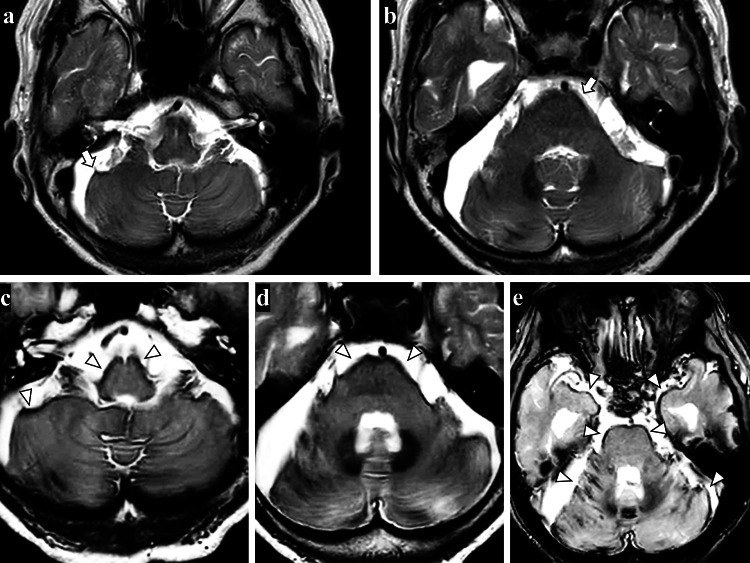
Pre- and postoperative brain MRI of the lumbar subarachnoid-peritoneal (L-P) shunt at the age of 78 years (a, b) and 80 years (c, d). (a, b) Preoperative axial T2-weighted MRI showing hemosiderin deposits on the surface of the brainstem and cerebellum (arrows); (c, d) At two years after L-P shunt surgery, T2-weighted MRI showing the increased deposition of hemosiderin (arrowhead); (e) T2*-weighted MRI showing numerous hemosiderin deposition (arrowheads).
